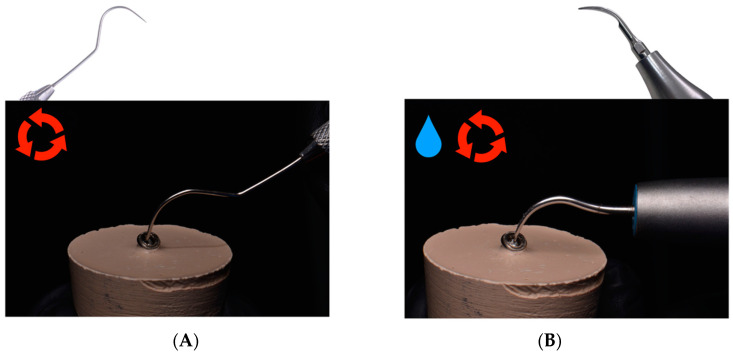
Conventional fractured abutment screw removal technique. (A) Exploration probe. (B) Ultrasound tip.
